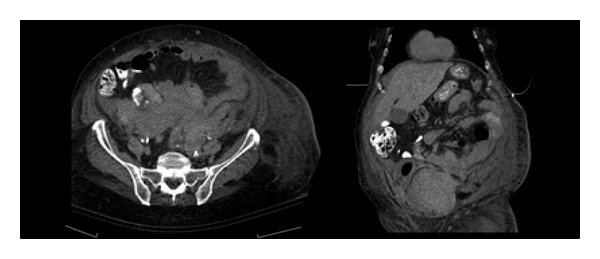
CT rectus sheath hematoma (Case  2)—a large retroperitoneal hematoma in the right posterior pararenal space, with an associated intramuscular hematoma in the right iliacus and right psoas muscle.
